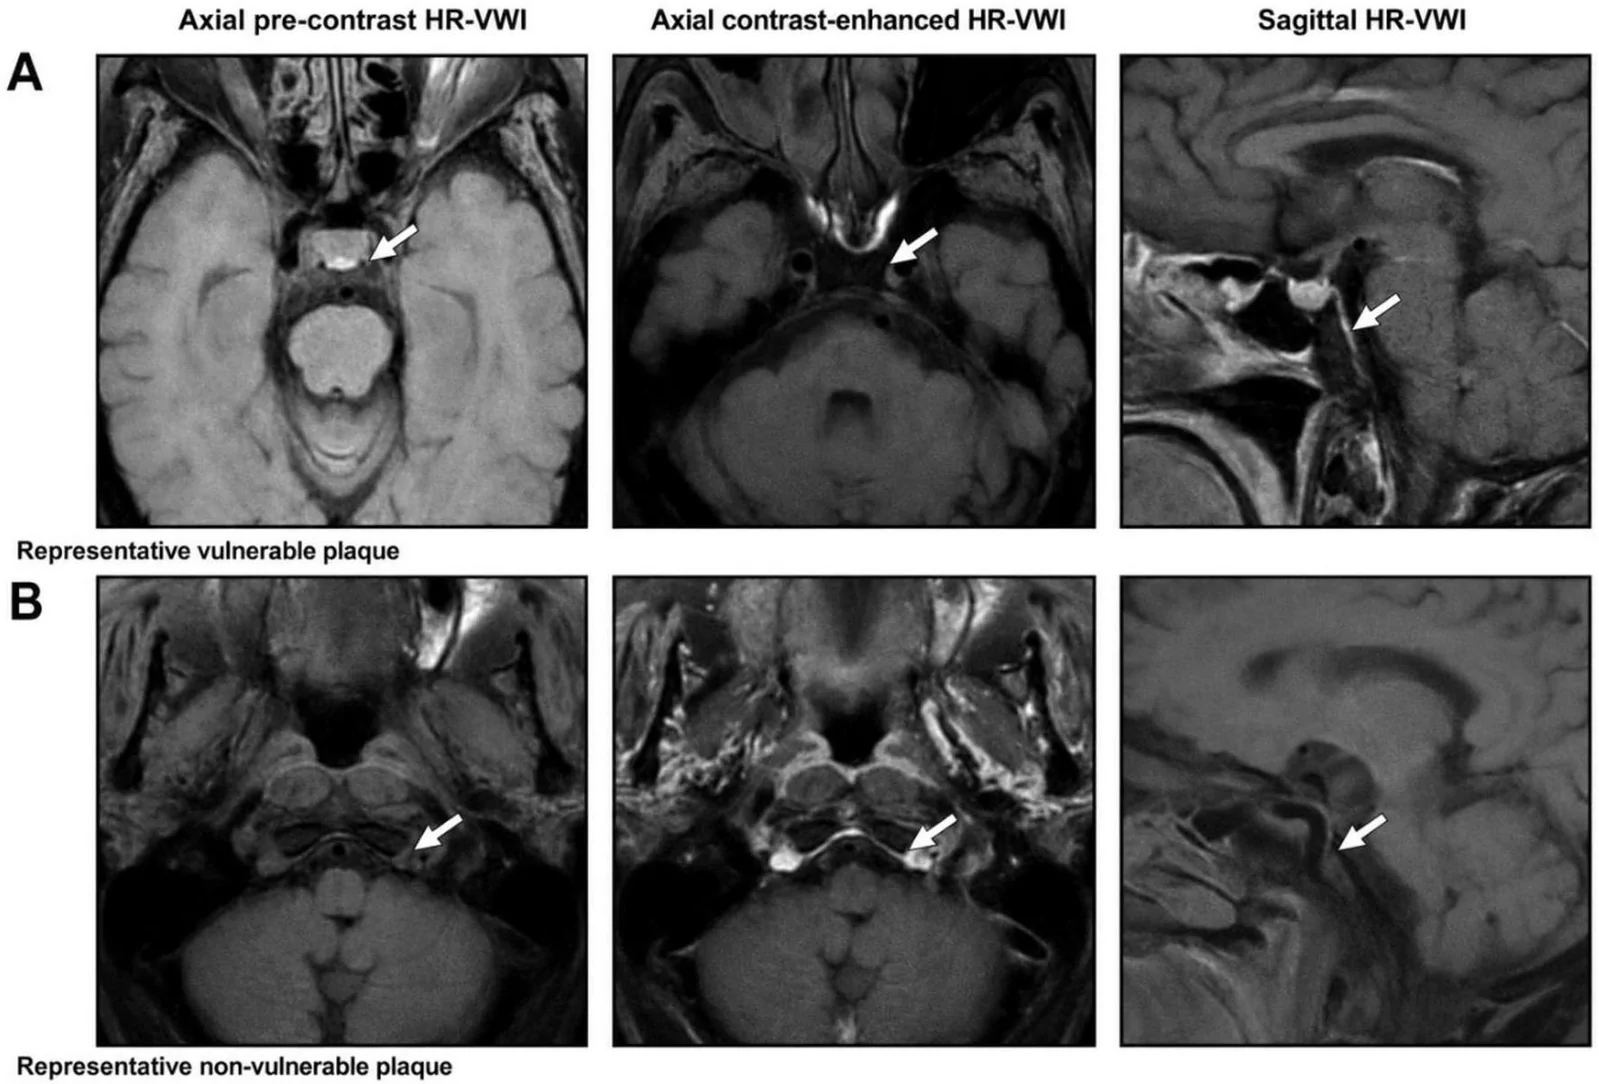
Vessel-wall MRI phenotypes of vulnerable and non-vulnerable vertebrobasilar plaques. (A1) Axial pre-contrast HR-VWI showing a representative vulnerable plaque. (A2) Axial contrast-enhanced HR-VWI showing plaque enhancement in the vulnerable plaque. (A3) Sagittal HR-VWI showing longitudinal localization of the vulnerable plaque. (B1) Axial pre-contrast HR-VWI showing a representative non-vulnerable plaque. (B2) Axial contrast-enhanced HR-VWI showing absent or mild enhancement in the non-vulnerable plaque. (B3) Sagittal HR-VWI showing longitudinal localization of the non-vulnerable plaque.
